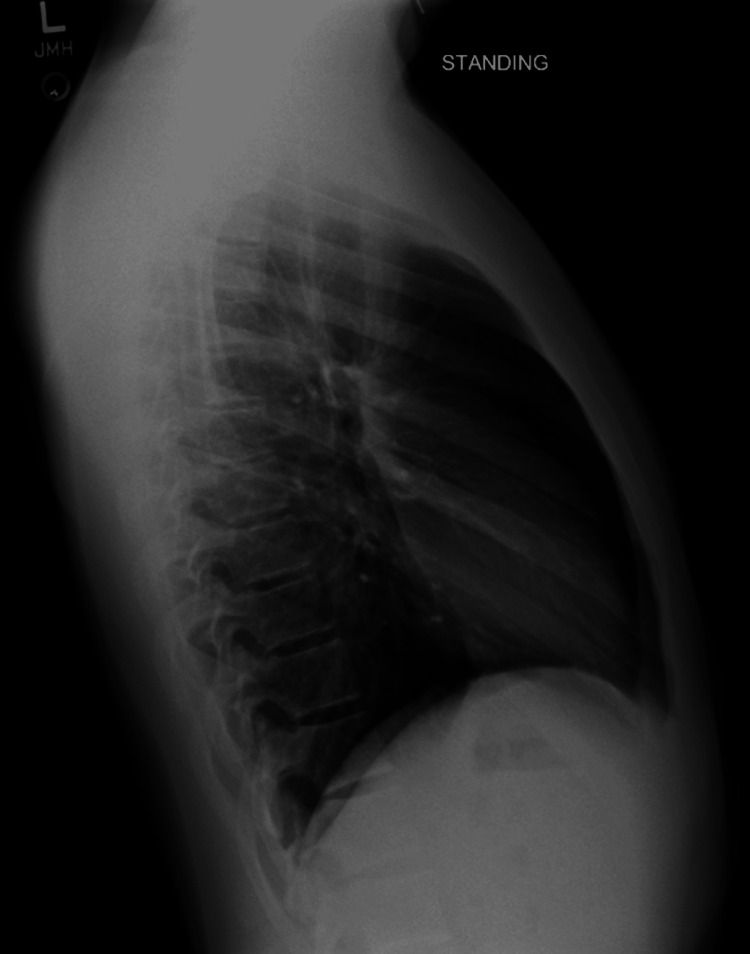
Lateral chest X-ray: one week follow up Normal reading
